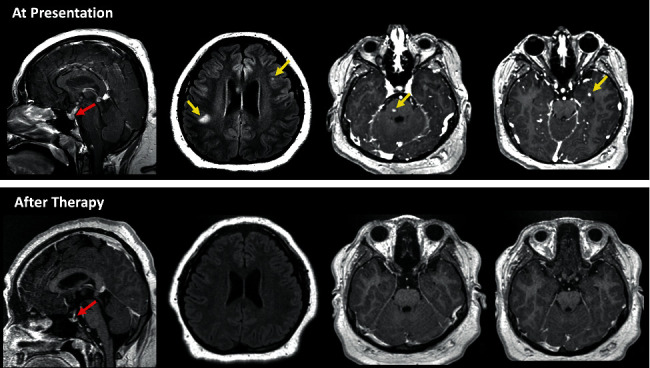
XD response to 2CdA on neuroimaging. MRI brain at presentation and comparison imaging after six cycles of 2CdA. Abnormal soft tissue at the pituitary stalk and extending into pituitary tissue has resolved after therapy (red arrows). Note that additional lesions in the supratentorial brain (yellow arrows) have also resolved after therapy.
